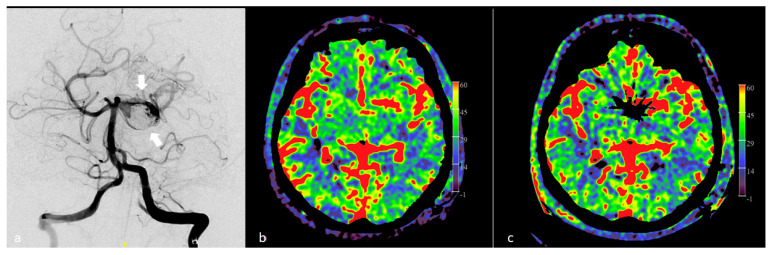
Intraprocedural FD CT PBV imaging in a case of PVO due to insufficiently treated dissecting P1–P2 aneurysm by stent assisted coiling. (a) DSA in AP view demonstrates the partially coiled dissecting aneurysm (white arrows). (b) Pre PVO FD CT PBV mapping demonstrates symmetric PBV maps. (c) Post PVO FD axial CT PBV MIP reformation shows no asymmetry in PBV values, suggesting the patient’s toleration to the PVO.
